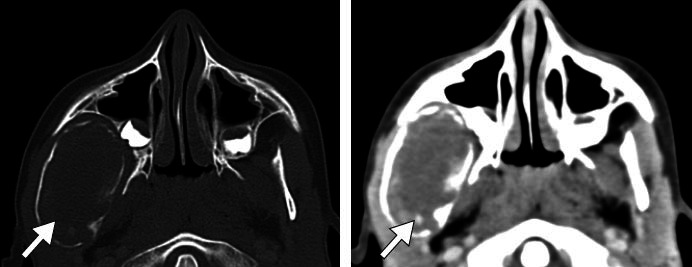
Ossifying fibroma in an 8-year-old male patient. CT scans showing an
